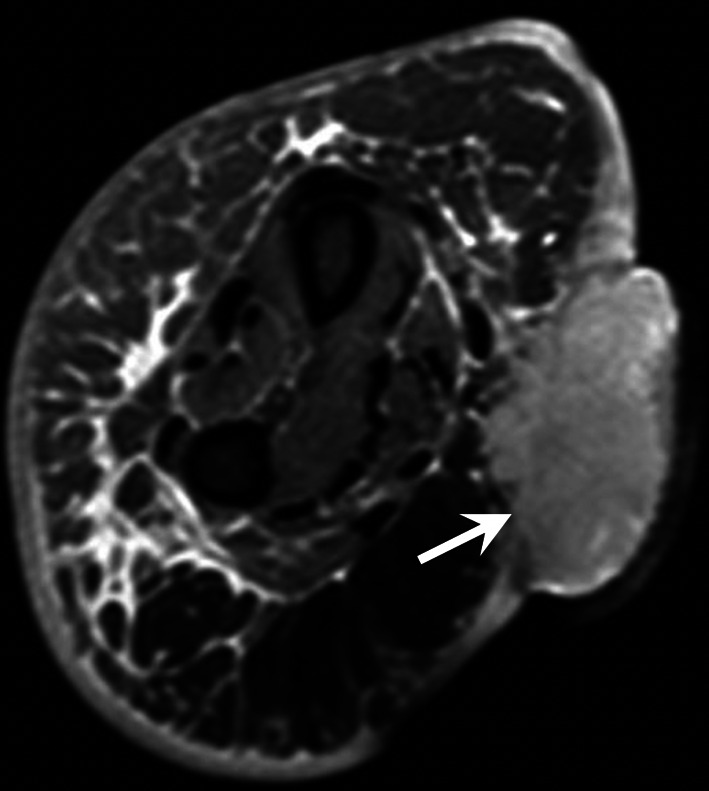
Stewart–Treves syndrome in a 78-year-old woman with a history of mastectomy with axillary lymph node dissection for breast cancer and chronic lymphoedema. Axial T2-weighted MR image showing a skin exophytic tumour developing at the palm of the wrist corresponding to an angiosarcoma (arrow). Note also the subcutaneous infiltration of soft tissue with a honeycomb pattern and the thickening of dermis indicating a chronic lymphoedema
